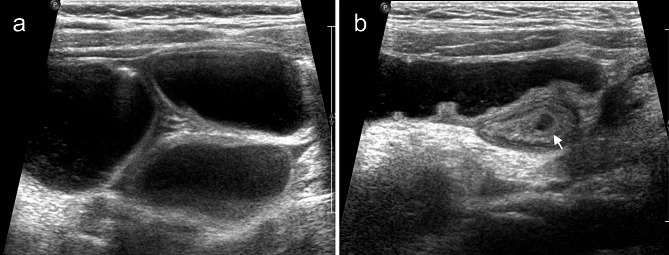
Representative imaging scans of a 7-year-old male patient presenting vomiting and abdominal pain. The ultrasound scan visualized Mekel’s diverticulum (white arrow), dilation of multiple segments of the small intestine and fluid accumulation in the abdominal cavity. Postoperative diagnosis of an IAH caused by Meckel’s diverticulum banding and secondary intestinal obstruction was made.
